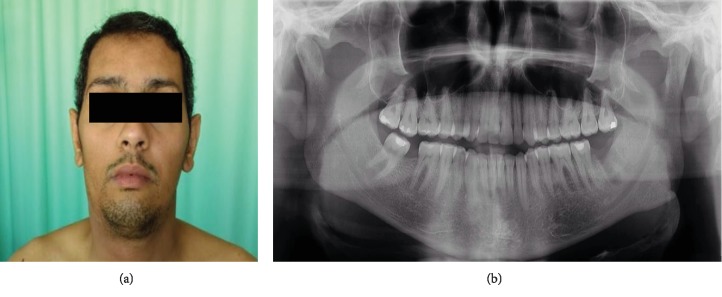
(a) Initial clinical aspect showing swelling in the submandibular region. (b) Panoramic radiograph taken after removal of the left mandibular third molar.
